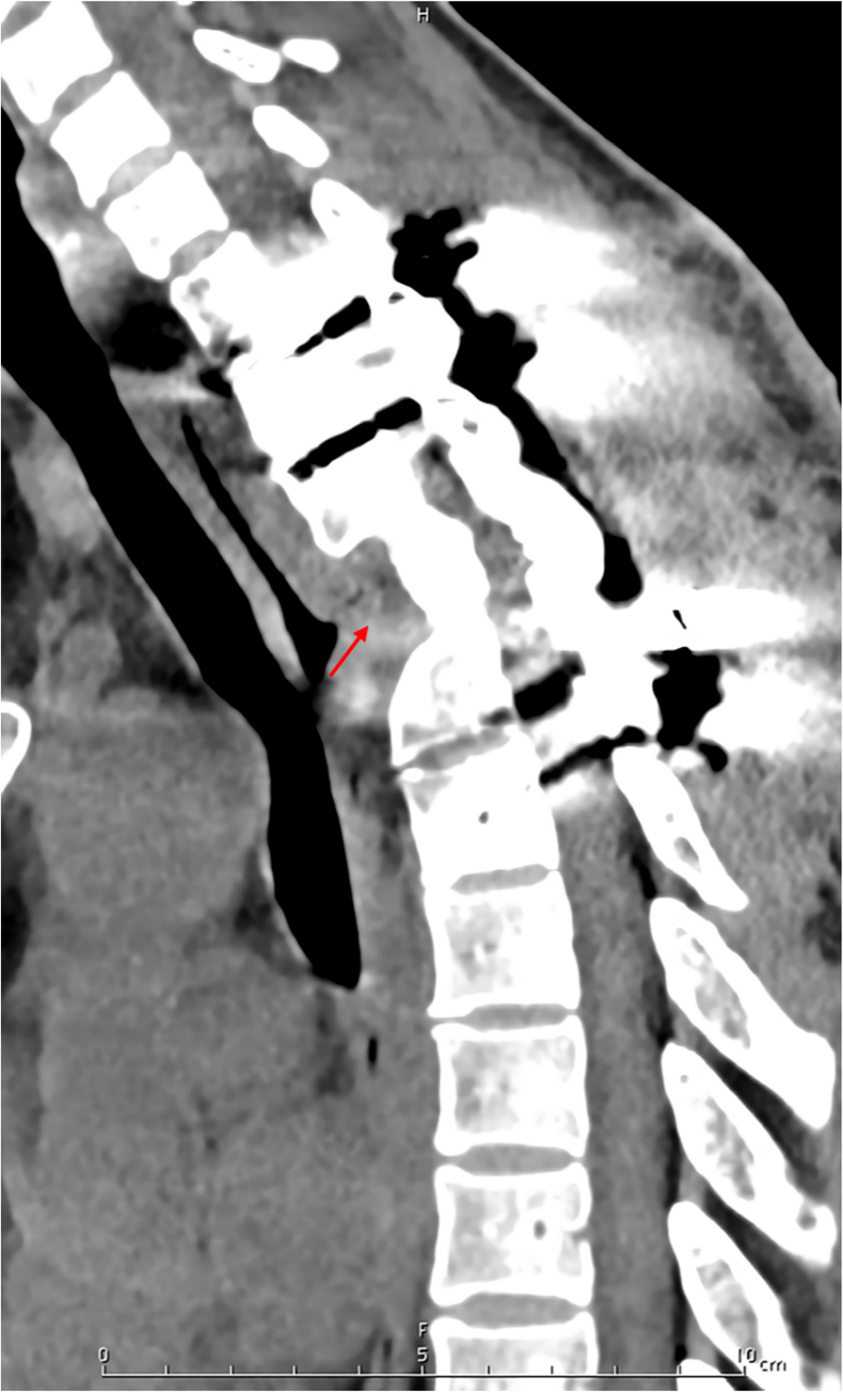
18 months after operation, CT examination showed that T2–4 had complete osseous fusion without dead bone existed
